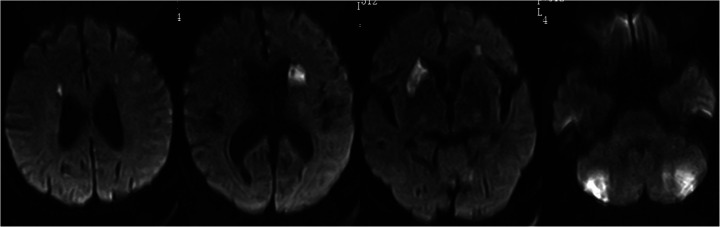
MRI DWI shows multiple acute infarctions in the bilateral periventricular regions, basal ganglia, and cerebellum, with a few lacunar infarctions in the bilateral frontal and parietal lobes.
